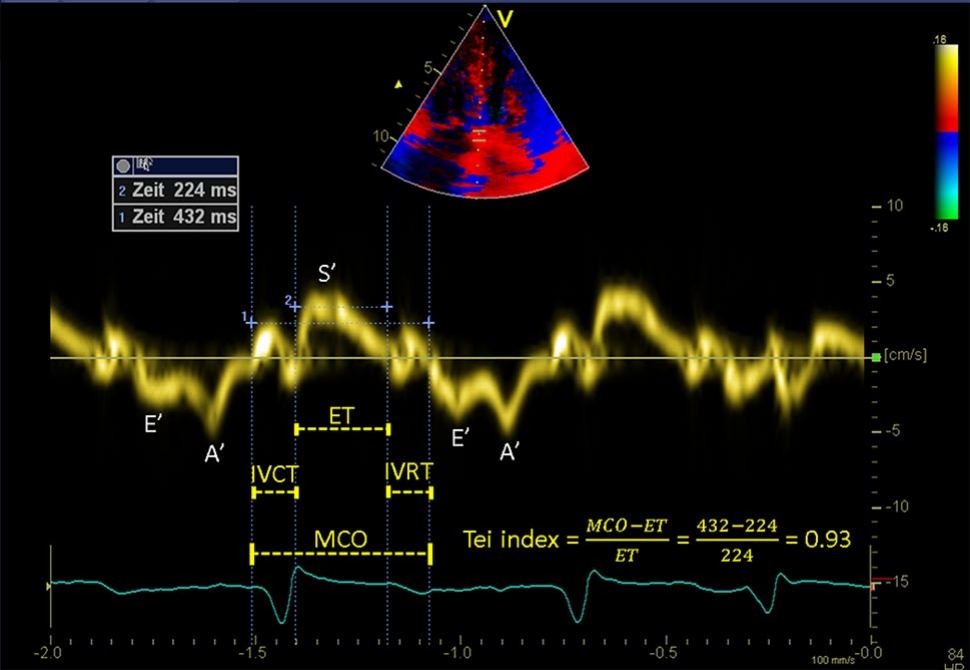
Example of a Tissue Doppler derived Tei index. Time intervals of left ventricle are measured with tissue Doppler imaging and the Tei index is calculated by the formula: (MCO-ET)/ET. *MCO* mitral valve closure-to-opening time, *ET* ejection time, *IVCT* isovolumetric contraction time, *IVRT* isovolumetric relaxation time, *S*′ peak systolic septal mitral annular velocity, *E*′ peak early-diastolic septal mitral annular velocity, *A*′ peak late-diastolic septal mitral annular velocity
